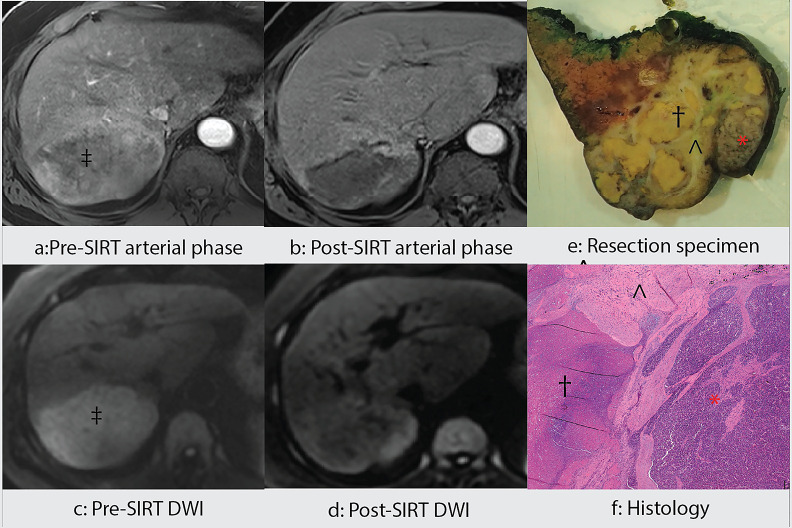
a–f Tumour (‡) demonstrating loss of arterial enhancement post SIRT (b) but persistent abnormal signal on DWI medially (d); pathological assessment of this region identified focus of residual tumour (*) interspersed with fibrosis (^) and tumour necrosis (†)
